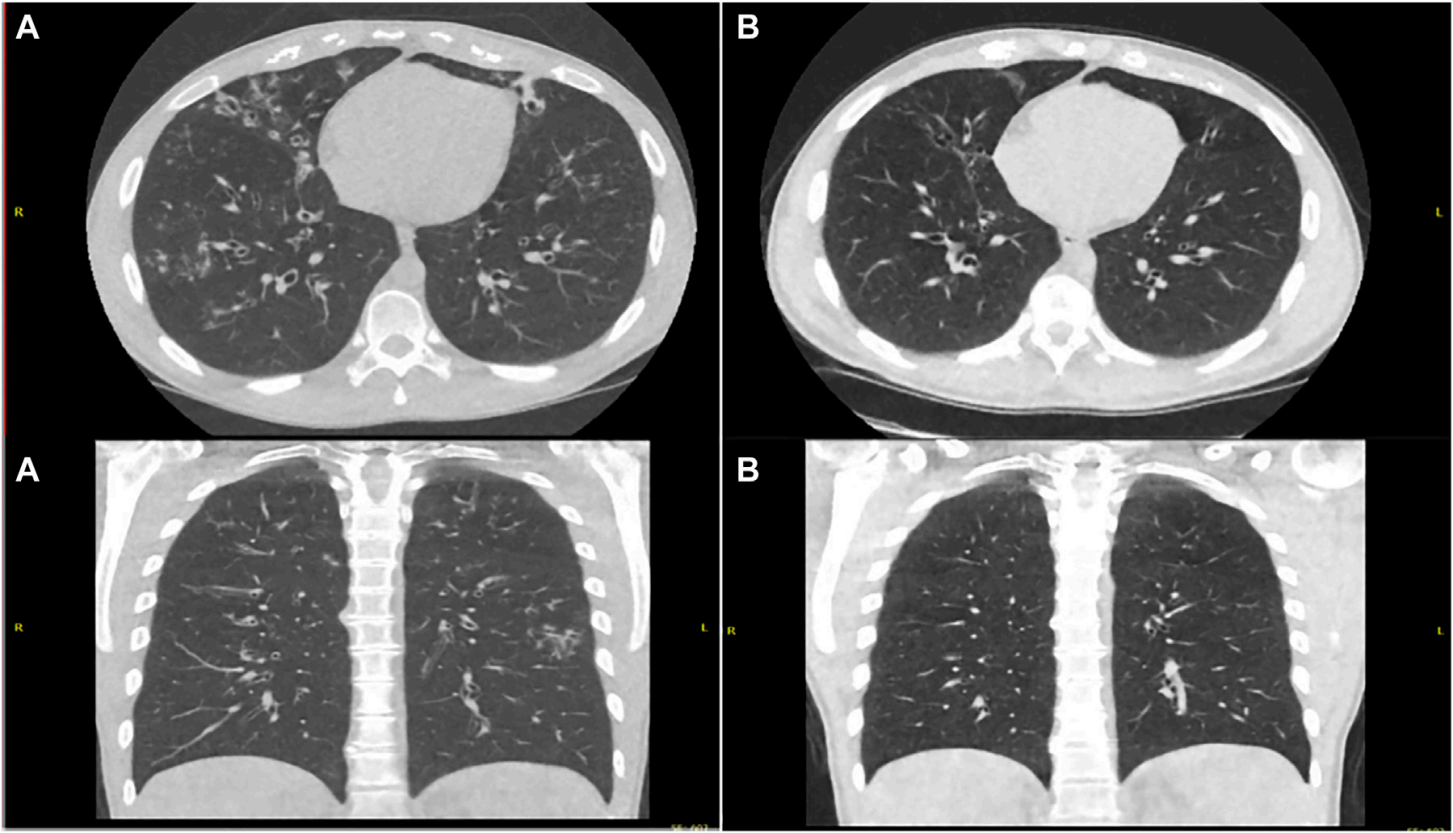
Ultra-Low Dose CT Thorax pretreatment (A) and post modified dose regimen (B) showing reduced burden of bronchiectasis, reduced caliber of bronchiectatic airways, decreased bronchial wall thickening and resolution of tree in bud opacification changes.
